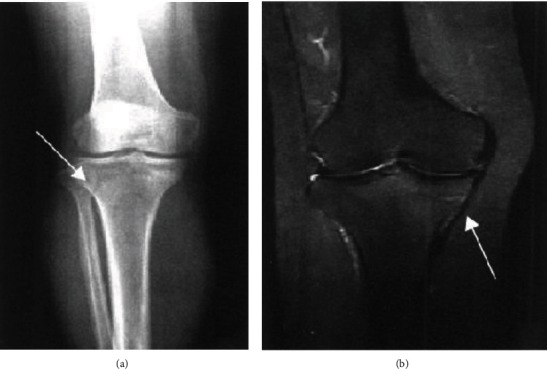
Stress fracture associated with tibial condyle motion based on (a) DR-positive film and (b) MRI coronal radiograph.
